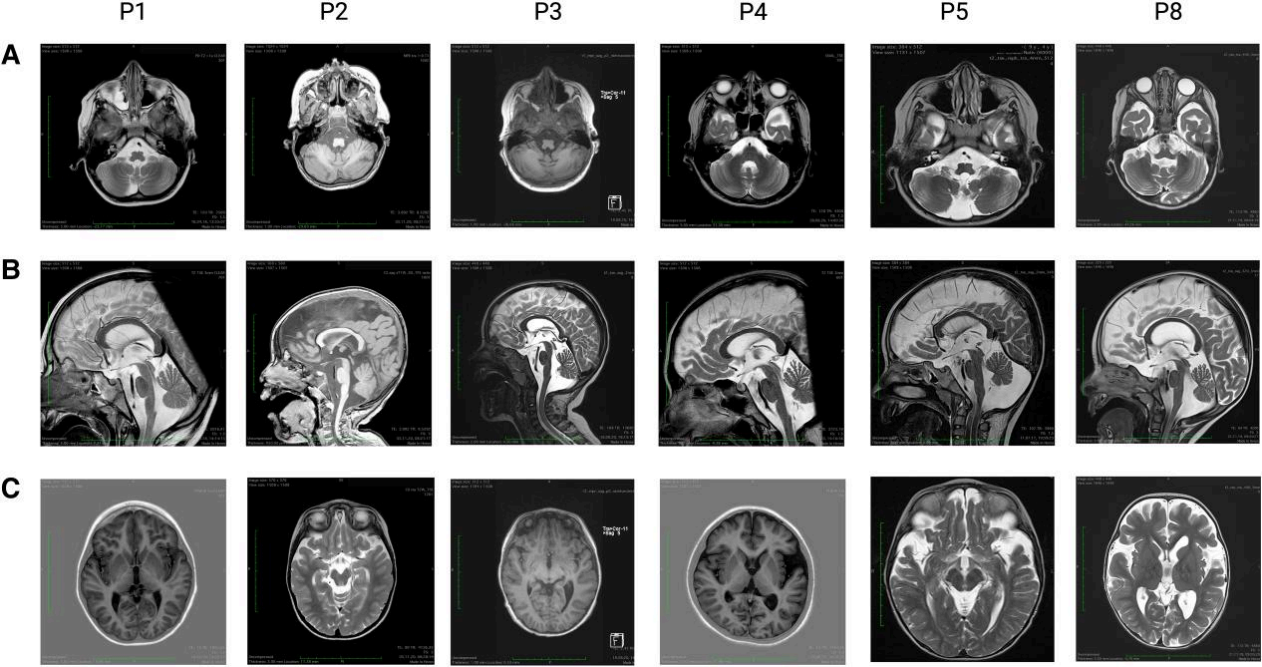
Homozygosity for the p.C112Wfs*11 SOD1 variant (C112X^Hom^) is associated with distinct cranial MRI alterations. Images display coronal and sagittal MRI scans from C112X^Hom^ patients 1, 2, 3, 4, 5, and 8. (A) Atrophy of the cerebellar vermis was seen to varying degrees in all analyzed patients. In those individuals who underwent serial imaging studies, the findings progressed over time (Table 2). (B) Brain stem atrophy was also observed in all 6 patients. (C) In 5/6 patients, some fronto-temporal atrophy was found, which did not progress visibly with age (range of observation period from 9 months to 12 years) in the three patients with serial studies.
